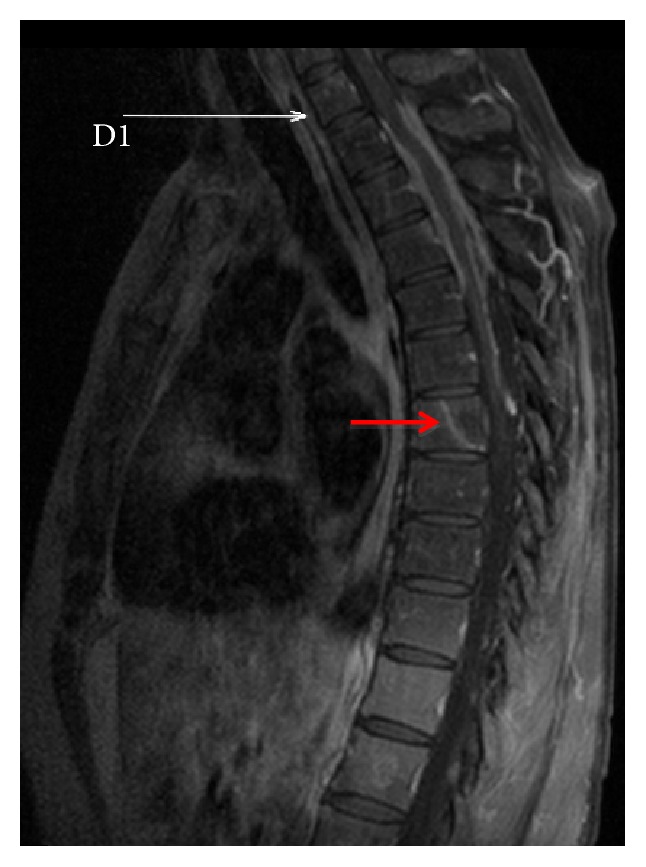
Sagittal gadolinium-enhanced T1-weighted Turbo Spin Echo Fat Sat MR image. The dorsal spinal cord is deformed and compressed by a sleeve of enhancing tissue, centered at the T2-T3 levels and extending between T2 and T7. The posterior body of T7 is infiltrated by a blastic lesion (hypointense on T1, T2, and STIR sequences) with peripheral enhancement (red arrow) highly suggestive of a bone metastasis.
